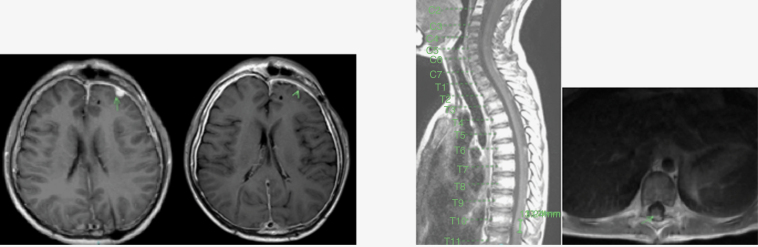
Representative MRI images from patients 5 and 6. Patient 5 (left): Brain lesion prior to re-induction (left) and nearly resolved after 4 cycles (right). Patient 6 (right): T10 lesion prior to re-induction (left) and nearly resolved “plaque” after 4 cycles (right).
